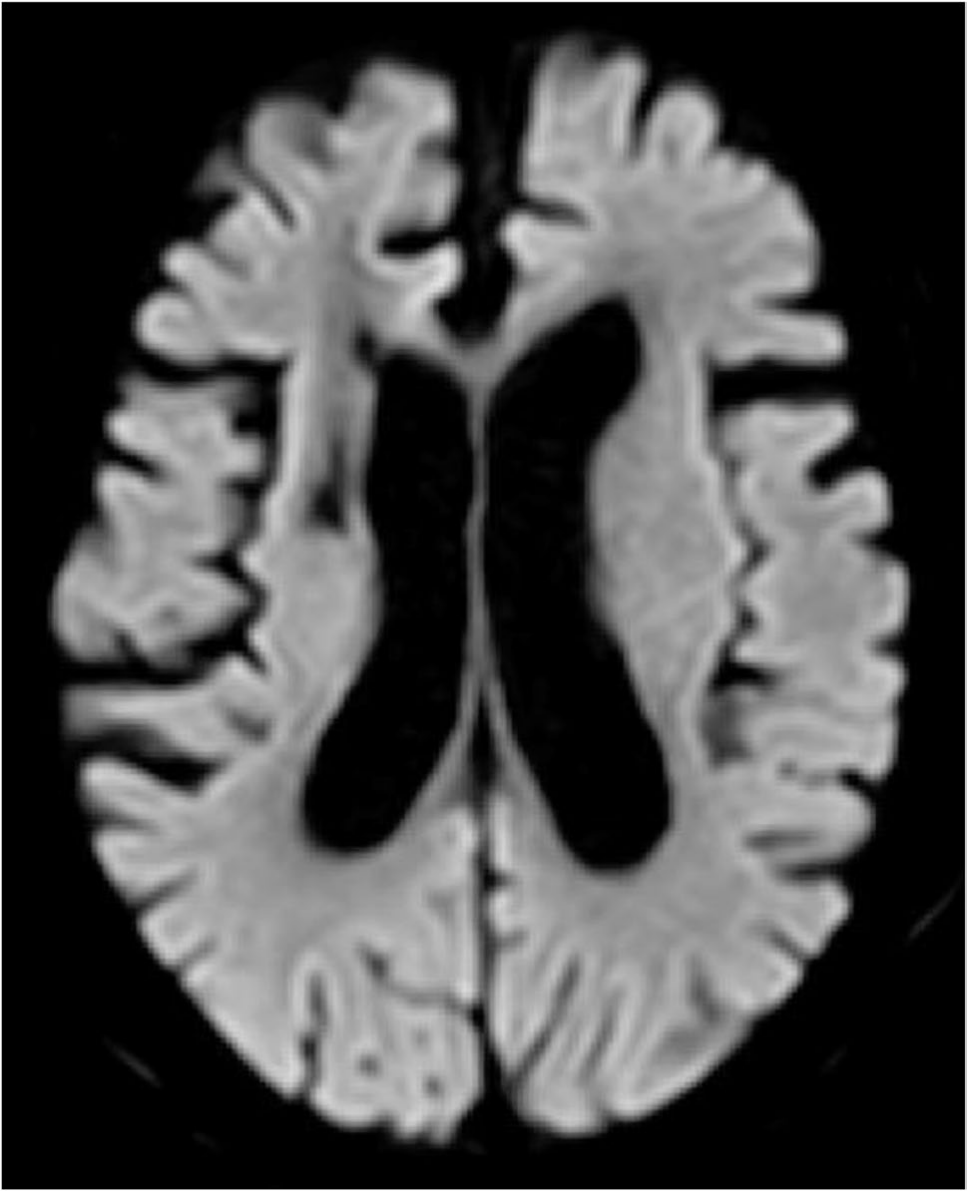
MRI in 2 months after surgery. MRI showing no new infarction; old infarct in right hemisphere only. MRI: magnetic resonance imaging
